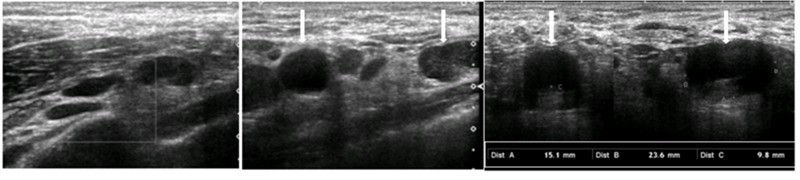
Ultrasound left axilla Enlarged hypoechoic left axillary lymph nodes (white arrows) showing cortical thickening with compressed/absent hilum.
